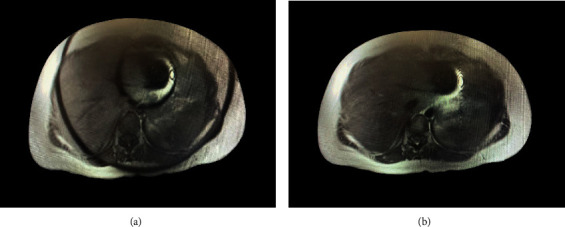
(a, b) MRI images of the mass.
